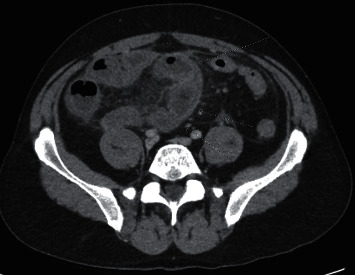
Axial CT image with IV contrast demonstrating inflammatory changes of the distal ileum. The bowel wall is thickened and edematous with surrounding fat stranding.
